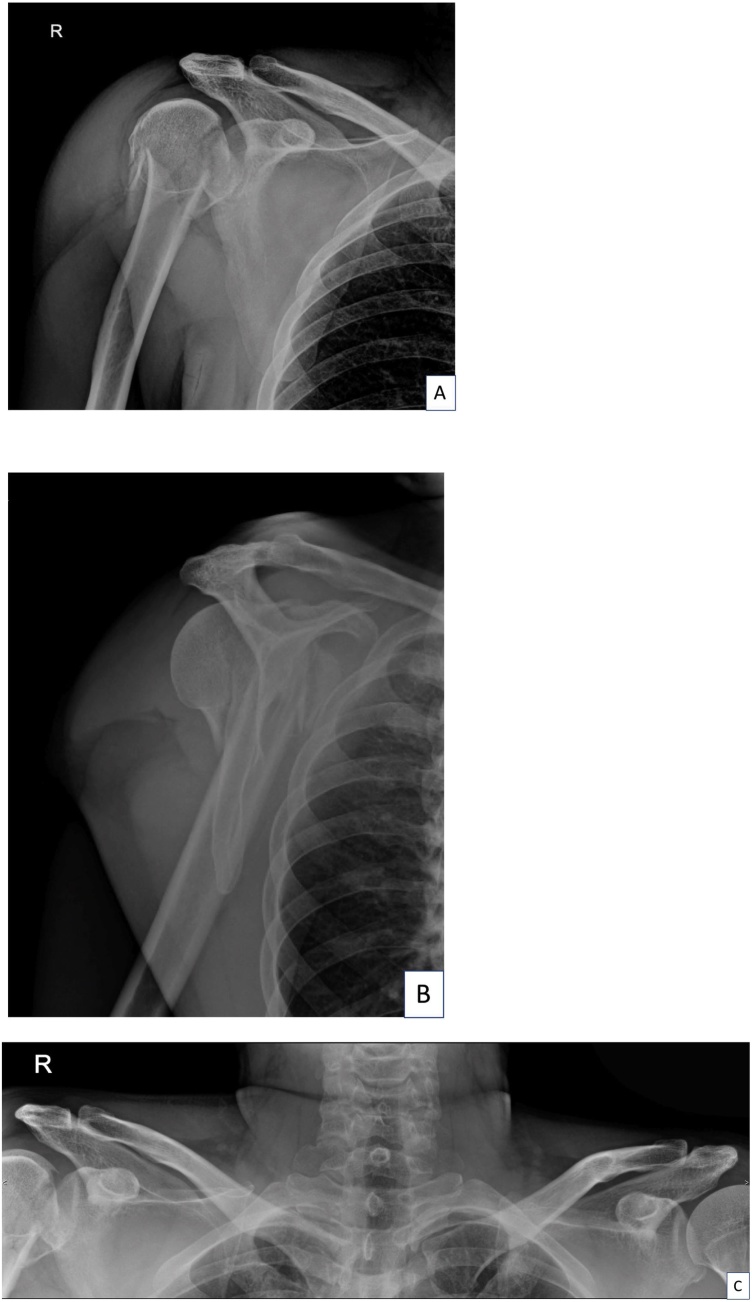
The initial radiographic imaging: (A) anteroposterior view of right shoulder, (B) transcapular view of right shoulder and (C) anteroposterior view of both clavicles.
